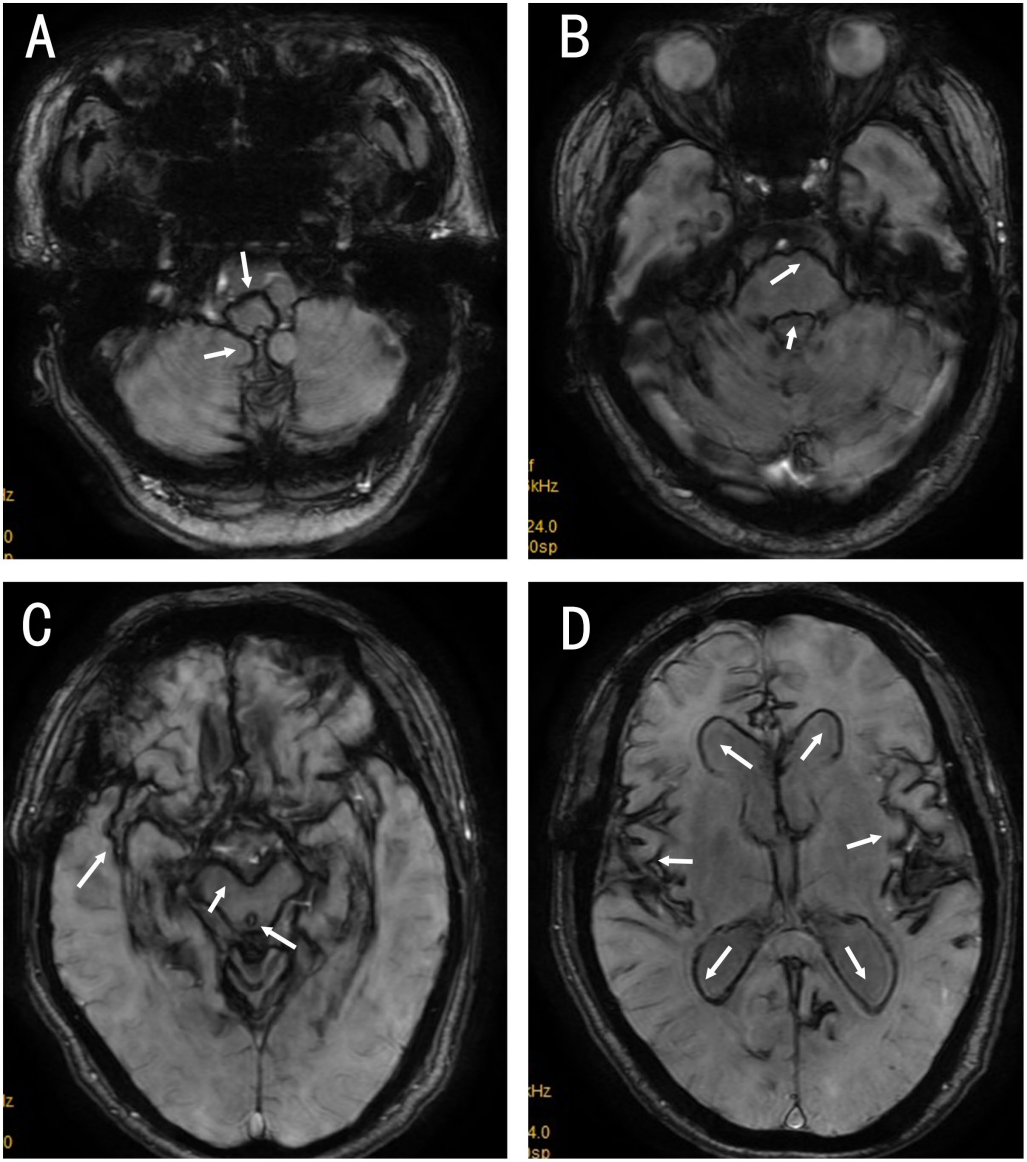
Non-contrast MRI (A–D). T2-weighted imaging (T2WI) shows linear hypointensity along the pial and periventricular surfaces, predominantly infratentorial. (A) Linear hypointensity around the medulla oblongata (black arrow) and over the cerebellar surface (white arrow(s)). (B) Linear hypointensity around the pons and along the medial temporal lobe (white arrow(s)), with bilateral temporal lobe white-matter hyperintensities. (C) Linear hypointensity around the midbrain (white arrow(s)). (D) Linear hypointensity around the Sylvian fissure (white arrow(s)), with enlargement of the lateral ventricles and cerebral atrophy.
